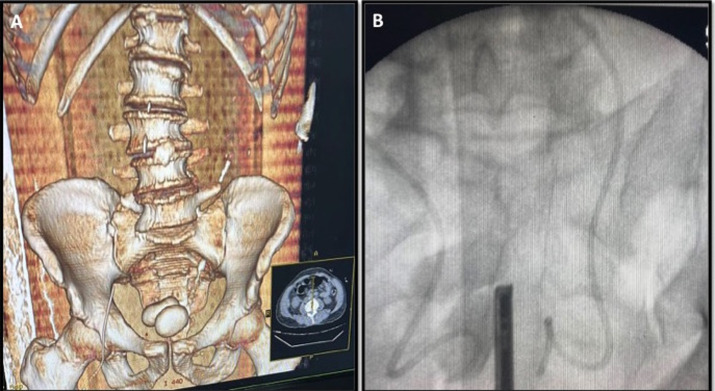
A) abdominal and pelvic CT scan showing two huge bladder stones; B) two huge bladder stones after the JJ probes have been placed using an image intensifier
